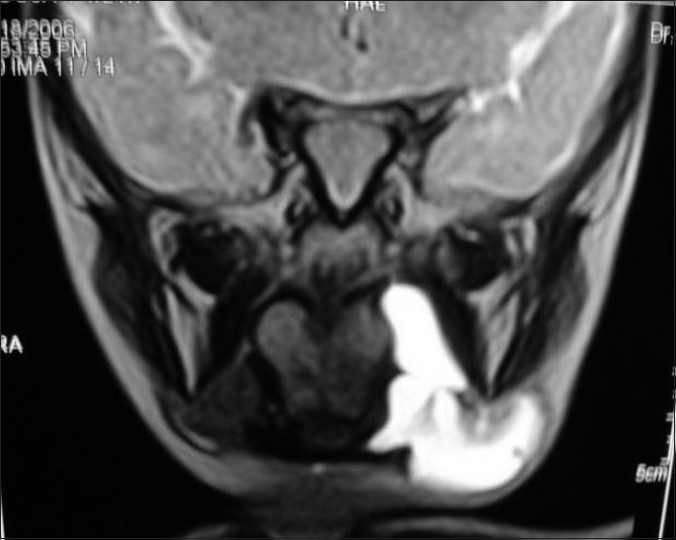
MRI showing cystic lesion on the left side in the sublingual region extending into the submental region, submandibular region, and the left parapharyngeal region up to the pharynx
